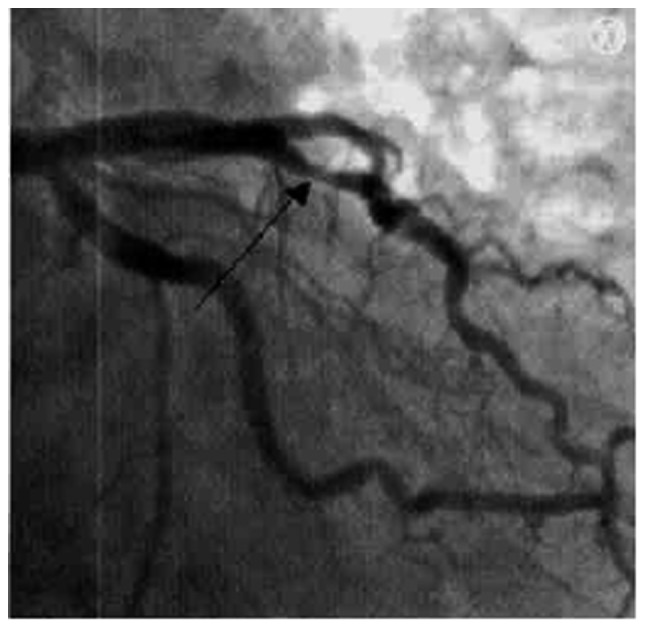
A myocardial bridge was observed in the proximal segment of the left anterior descending coronary artery by selective X-ray coronary angiography.
